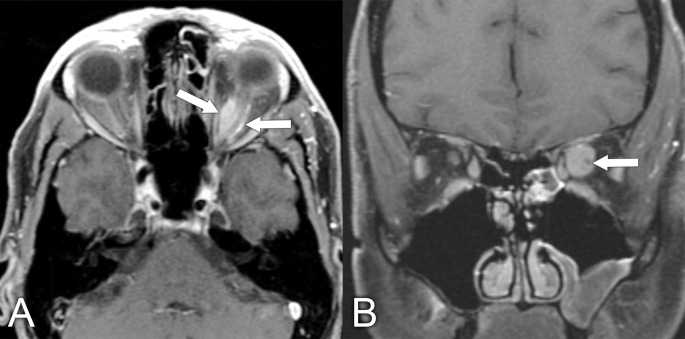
A 47-year-old woman presented with slowly progressive visual loss in the left eye. On exam, she had moderate visual loss with a relative afferent pupillary defect and a swollen left optic nerve head. (A) Axial T1 postcontrast MRI with fat saturation shows a lesion around the optic nerve with a “tram-track”-like appearance (arrows). (B) Coronal T1 postcontrast MRI with fat saturation in the same patient shows the enhancing mass surrounding the left optic nerve (arrow). The diagnosis, based on radiologic findings, was optic nerve sheath meningioma. The patient was treated with proton radiation therapy and experienced improvement in her visual function.
